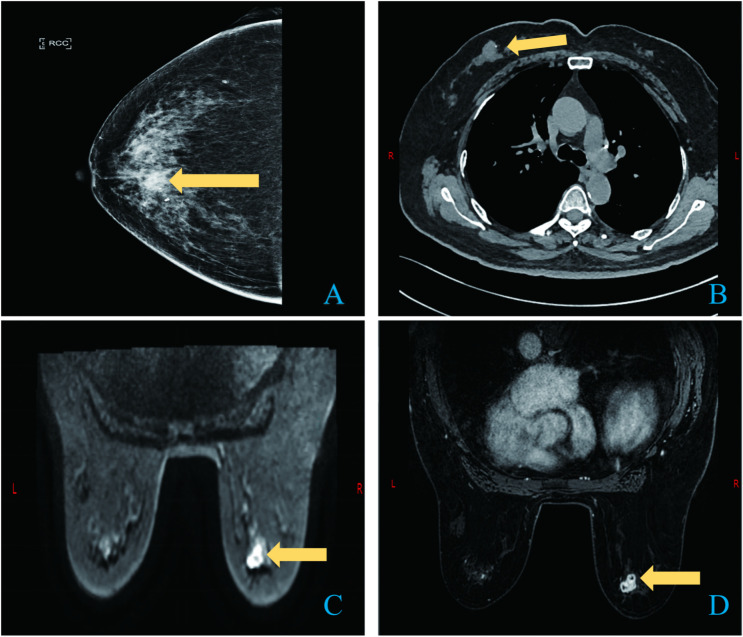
Multimodal imaging of the right breast lesion (A–D). (A) Mammography shows a nodular opacity in the upper quadrant of the right breast. (B) Non-contrast chest CT shows a right breast nodule with peripheral calcifications. (C) Breast MRI DWI shows a hyperintense lesion in the upper inner quadrant of the right breast. (D) DCE-MRI shows marked enhancement with a washout-type time–signal intensity curve. Yellow arrows indicate the lesion.
